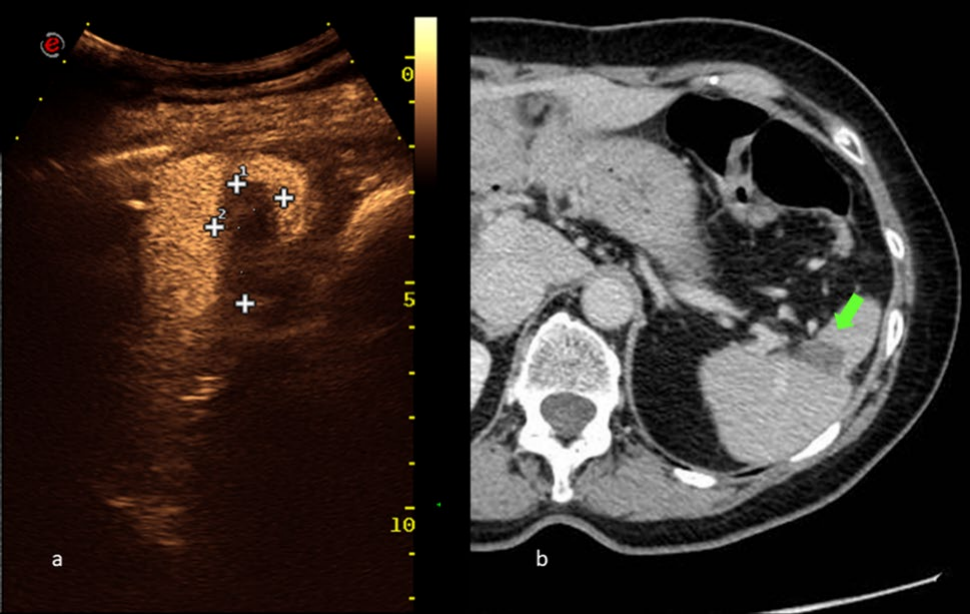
Contrast enhanced ultrasound (CEUS) and computed tomography (CT)-scan imaging at 6-months follow-up. The selected area at CEUS image (5a) shows an anechoic area corresponding to the necrotic area. Late stage-CT scan shows hypodense lesion, corresponding to the lesions treated by radiofrequency ablation in the spleen (pointed with arrow). Both the techniques show no residual tumor
